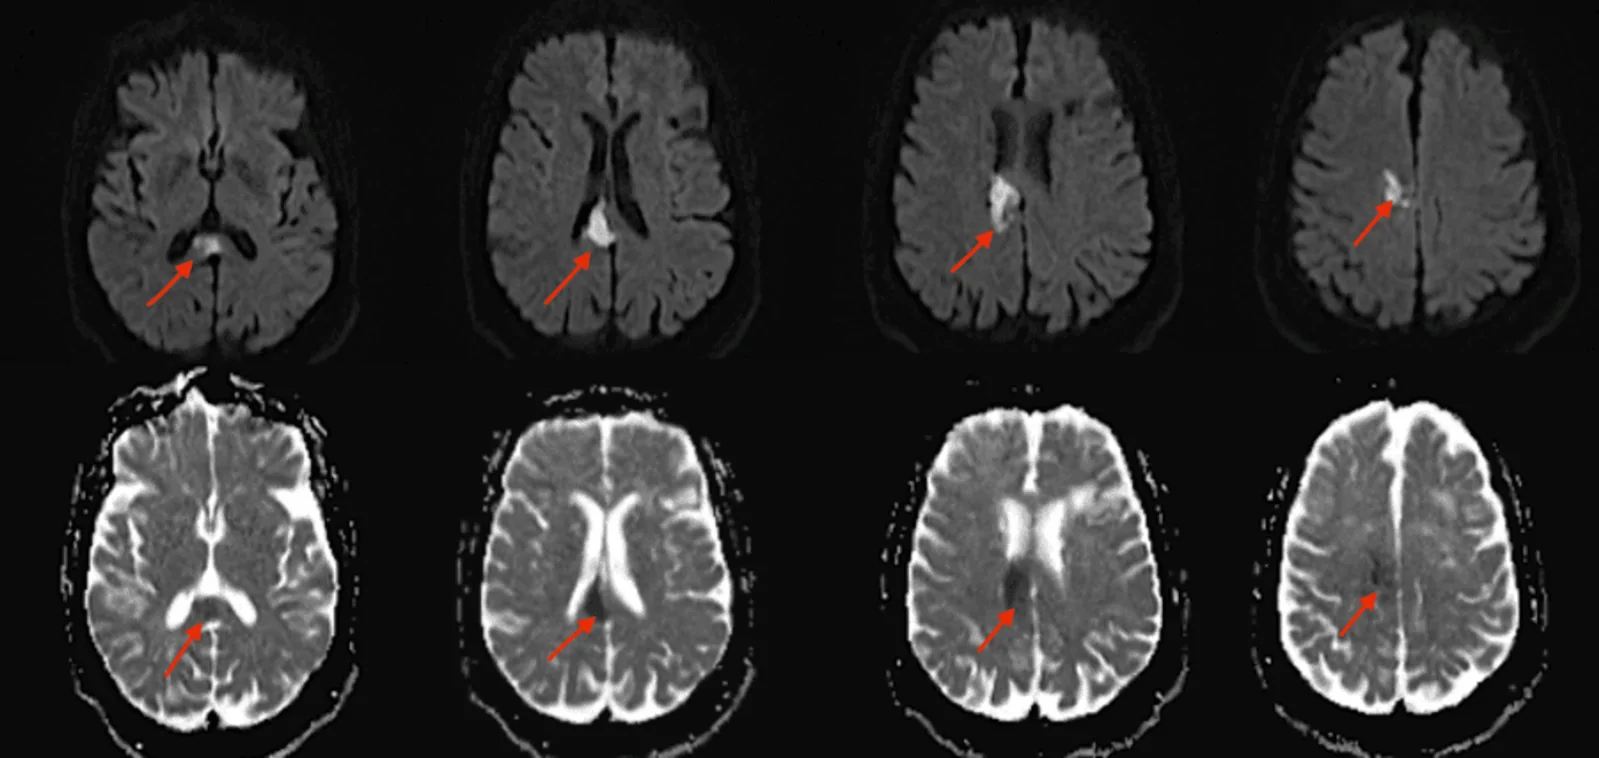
Acute callosal ischemic stroke on brain MRI axial DWI showing a hyperintense signal (red arrows, top row) with restricted diffusion on the ADC map (red arrows, bottom row), involving the posterior two-thirds of the body and the splenium of the CC MRI: magnetic resonance imaging, DWI: diffusion-weighted imaging, ADC: apparent diffusion coefficient, CC: corpus callosum
